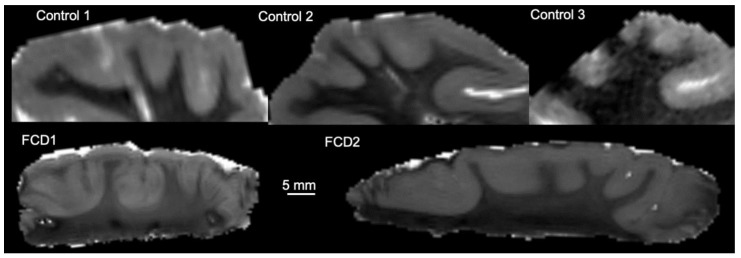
Representative greyscale MR images. Representative mean diffusivity (MD) images of the central slice of the medial temporal gyrus of the two FCD cases, as well as the 3 control postmortem hippocampi.
